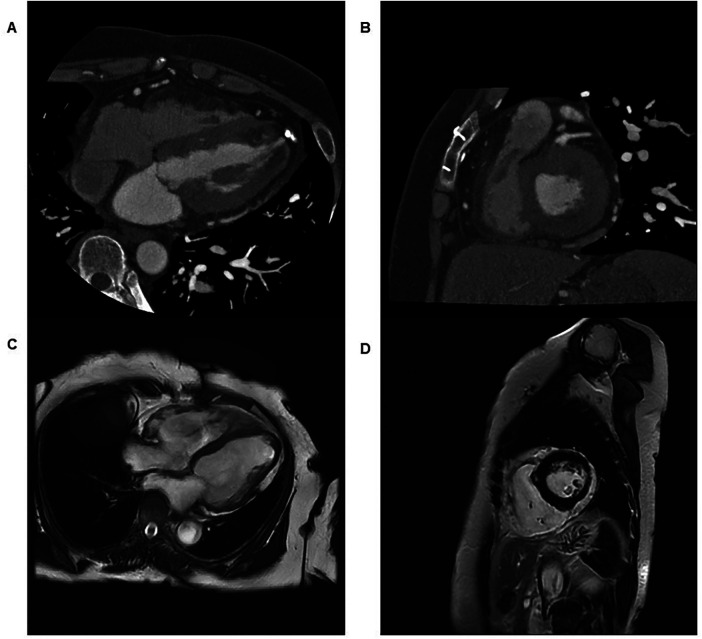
Multimodality imaging findings in two patients with arrhythmogenic cardiomyopathy. (A) Axial cardiac computed tomography image from Case 1 demonstrating fatty hypertrophy of the right ventricular free wall. (B) Axial cardiac computed tomography image from Case 1 demonstrating subepicardial fatty infiltration of the left ventricular apex, indicating left ventricular involvement. (C) Still frame captured from cardiac magnetic resonance cine imaging of Case 2 showing global right ventricular hypokinesia. (D) Short-axis cardiac magnetic resonance image from Case 2 demonstrating right ventricular wall thinning and akinesia. (See Supplementary Video S1 for the corresponding cine sequence.).
